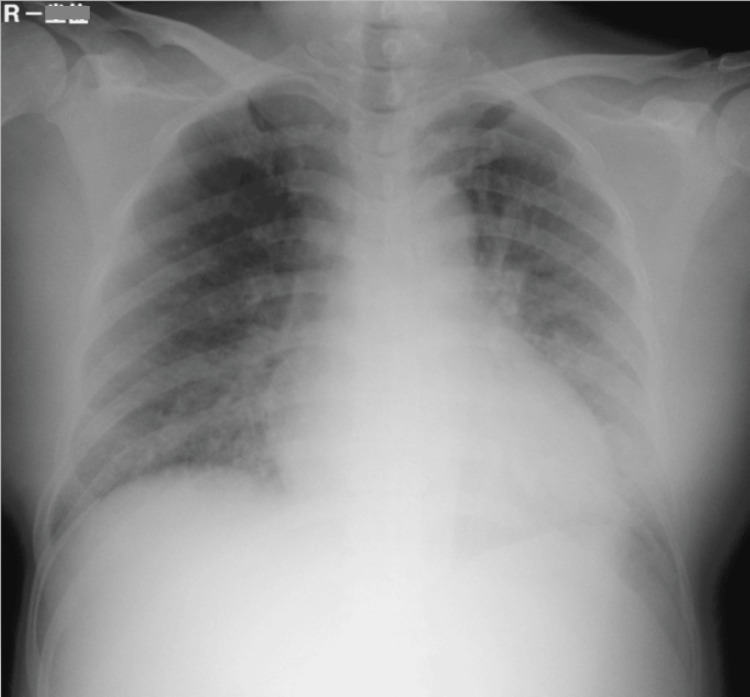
Chest X-ray shows bilateral opacities and butterfly shadow
